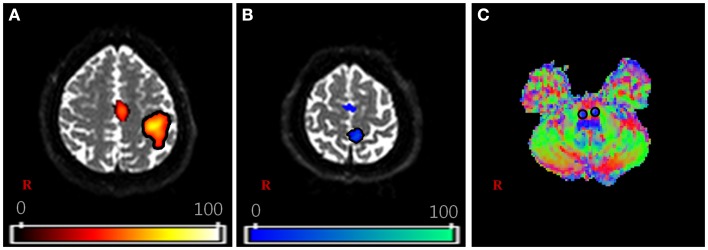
The region of interest (ROI) setting for fiber tracking. (A,B) are shown the activation area of functional MRI with finger and toe movement, respectively. (C) is shown the part of lower portion of the pons on the V1 image of diffusion-tensor image (DTI) dataset. The V1 image is the vector image for principle tensor direction and colors indicate the direction of vector (red: right–left, green: anterior–posterior, blue: superior–inferior). For each subject, the hand fiber tracts were extracted using two ROIs that were placed on the (A,C). The foot fiber tracts were used (B,C).
